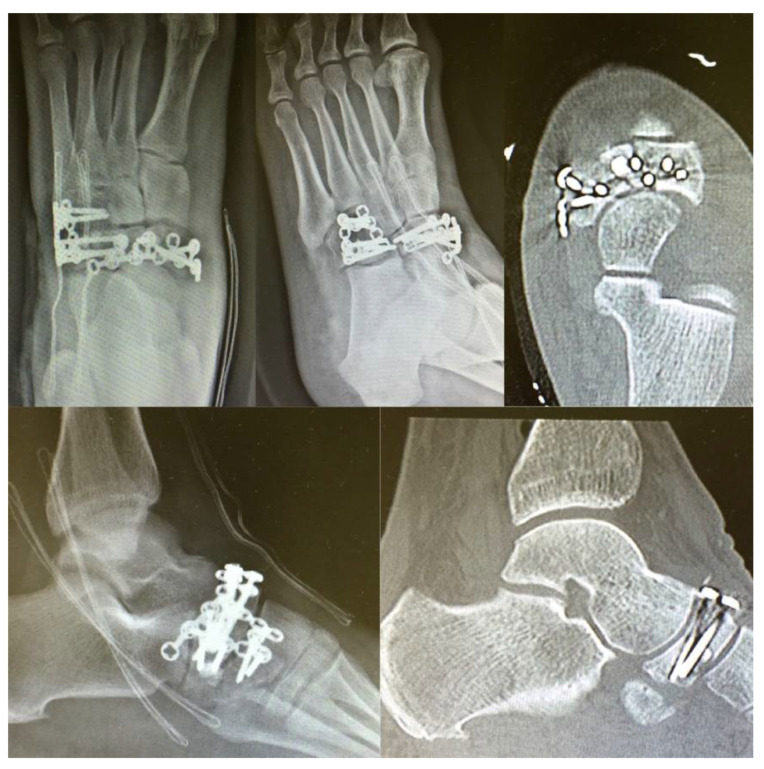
Postoperative X-rays imaging and CT scan.
